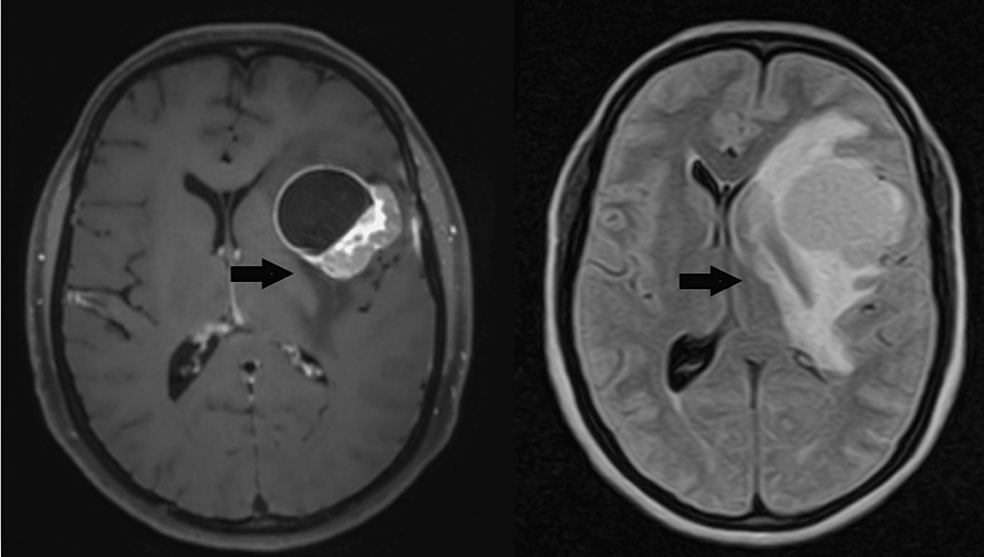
An axial post-contrast T1 image shows a large mass in the left insular region, demonstrating peripheral rind contrast enhancement with FLAIR hyperintense signals in the left anterior temporal lobe, left basal ganglia, and left frontal lobe (black arrows). FLAIR:  fluid attenuated inversion recovery
